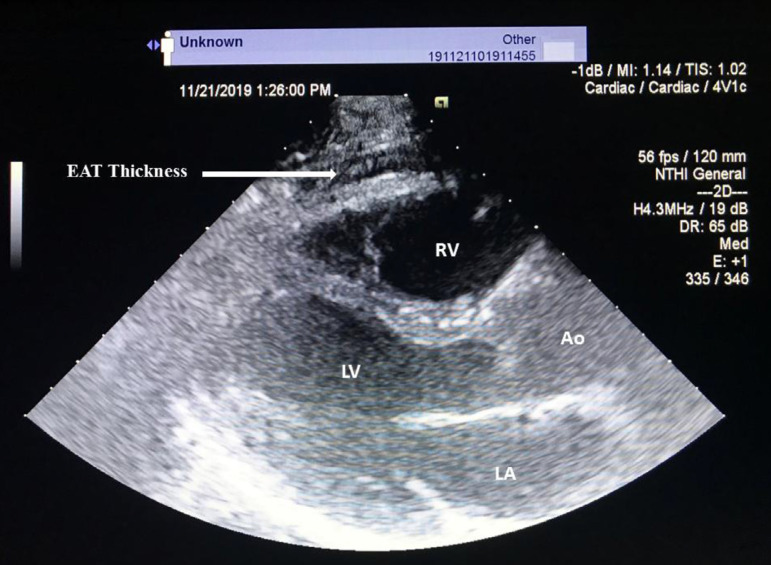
EAT was identified as an echo-free space between external myocardium and visceral pericardium from the parasternal long-axis view and was measured perpendicularly in front of the right ventricular free wall at end-diastole; AO=aorta; LA=left atrium; LV=left ventricle; RV=right ventricle
